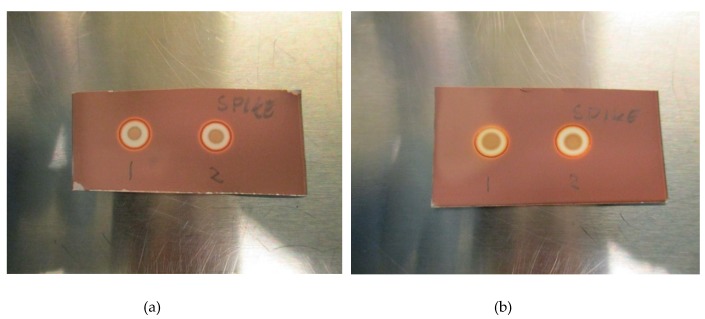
(a): Spike without antioxidant: (1) Matrix spiked with TBA, (2) [^18^F]PSMA-1007 spiked with TBA. (b): Spike with antioxidant: (1) Matrix spiked with TBA, (2) [^18^F]PSMA-1007 spiked with TBA.
